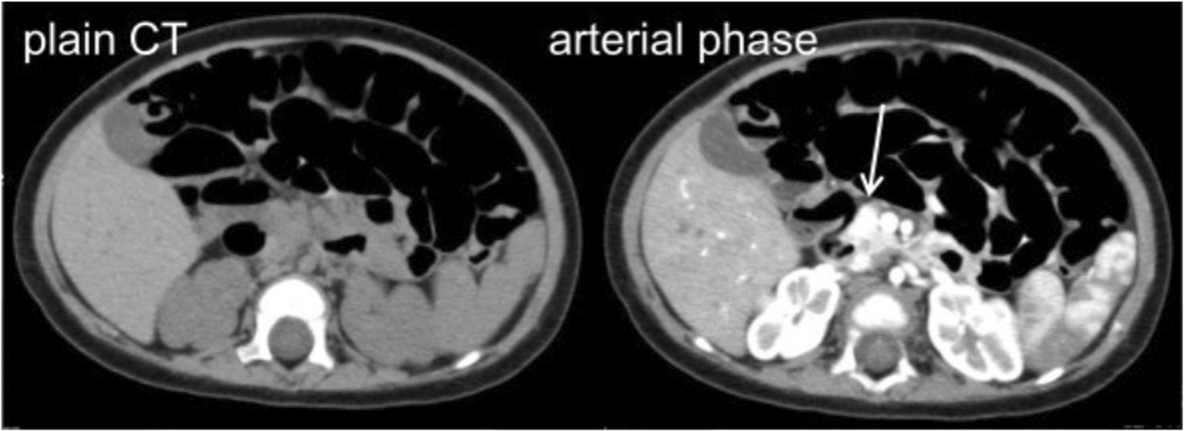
CT scan. A hyperenhancing nodule was identified on the arterial phase
